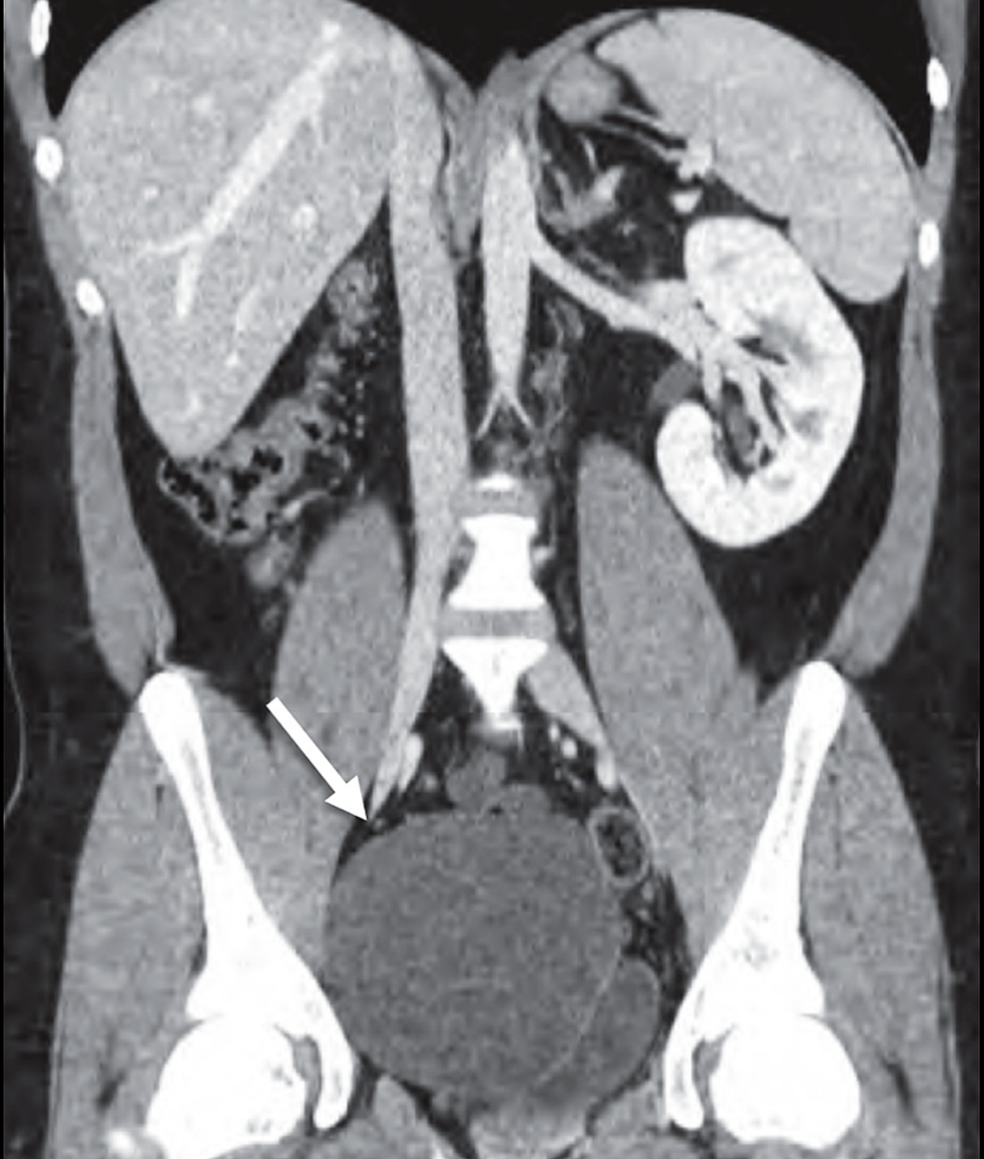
Coronal computed tomography image. Coronal computed tomography image of the abdomen showing a right-sided well-defined cyst in the pelvis (arrow) with an absent right kidney.
